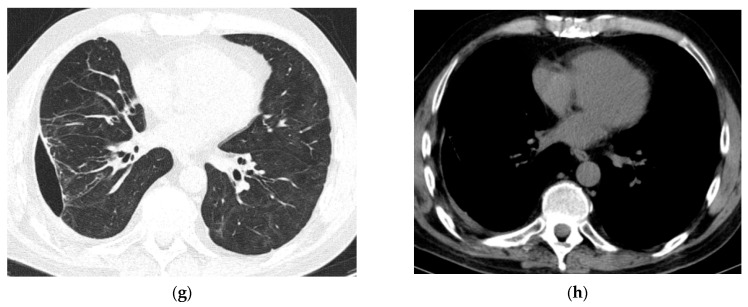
Radiologic evolution of case 1: Initial chest CT scan (axial and coronal plane) showing right lower lobe abscess prior to rupture into pleural space and post-COVID-19 infection infiltrates (a,b). CT scan after abscess rupture and prior to percutaneous drainage showing air cavity with dense fluid and hydro-aeric level occupying almost the entire right lung field; remaining right lung parenchyma with fibrotic changes, and a large lung abscess compressed at the hilum; small left pleural collection; small pericardial effusion (c,d). After the insertion of percutaneous ICD, CT scans follow-up reveals decreased volume of the residual cavity, thickened pleura and intracavitary drainage (e); slightly re-expanded right lung parenchyma; numerous ground-glass opacities (GGO), areas of fibrosis and passive atelectasis (e,f). After chest tube removal (290 days of drainage), minimal residual cavity without fluid and thin pleural fibrotic changes remained (g,h).
